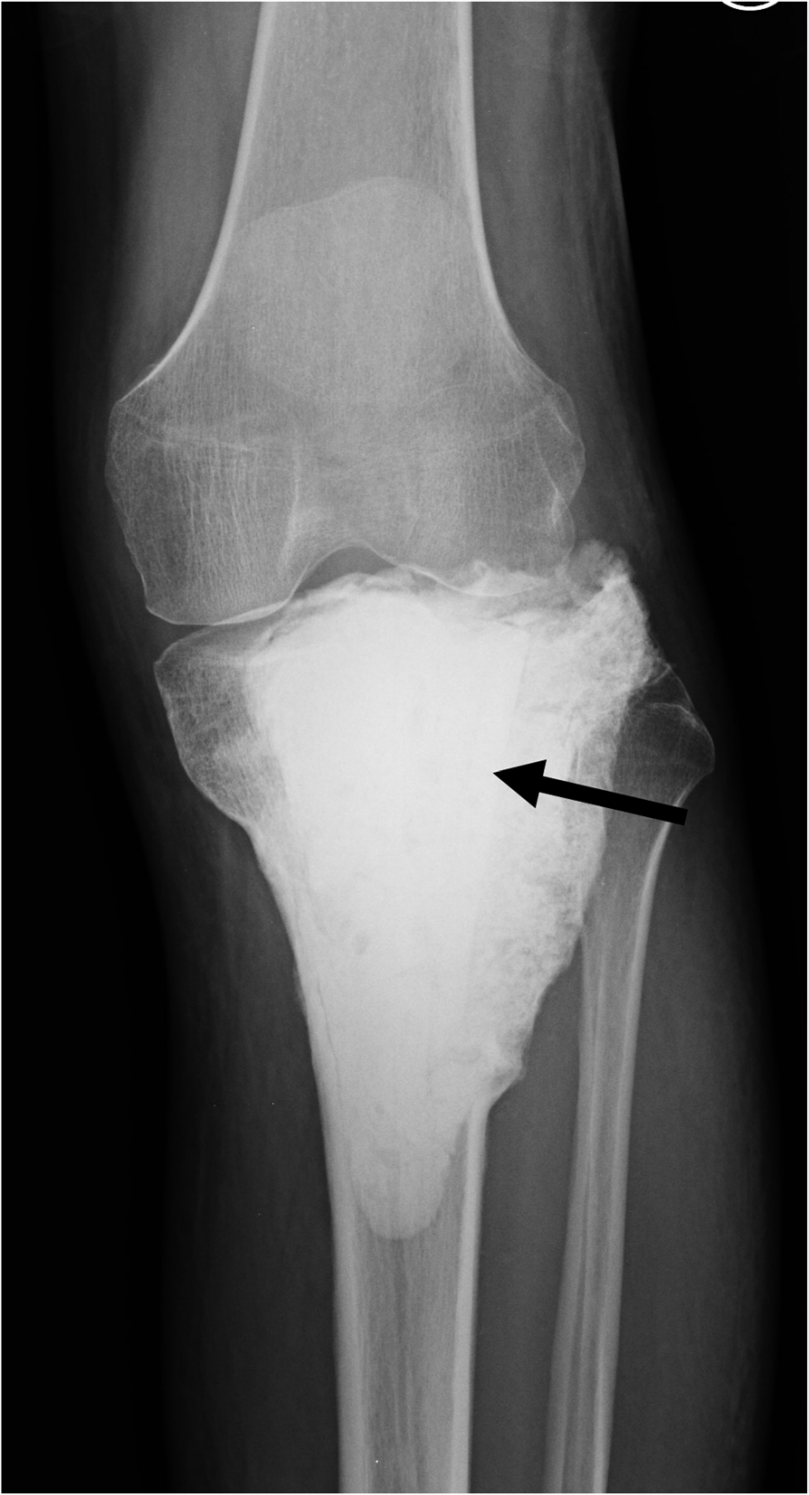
Radiography after cement filling following large cortical bone allograft insertion after curettage. The arrow indicates the large cortical bone allograft
